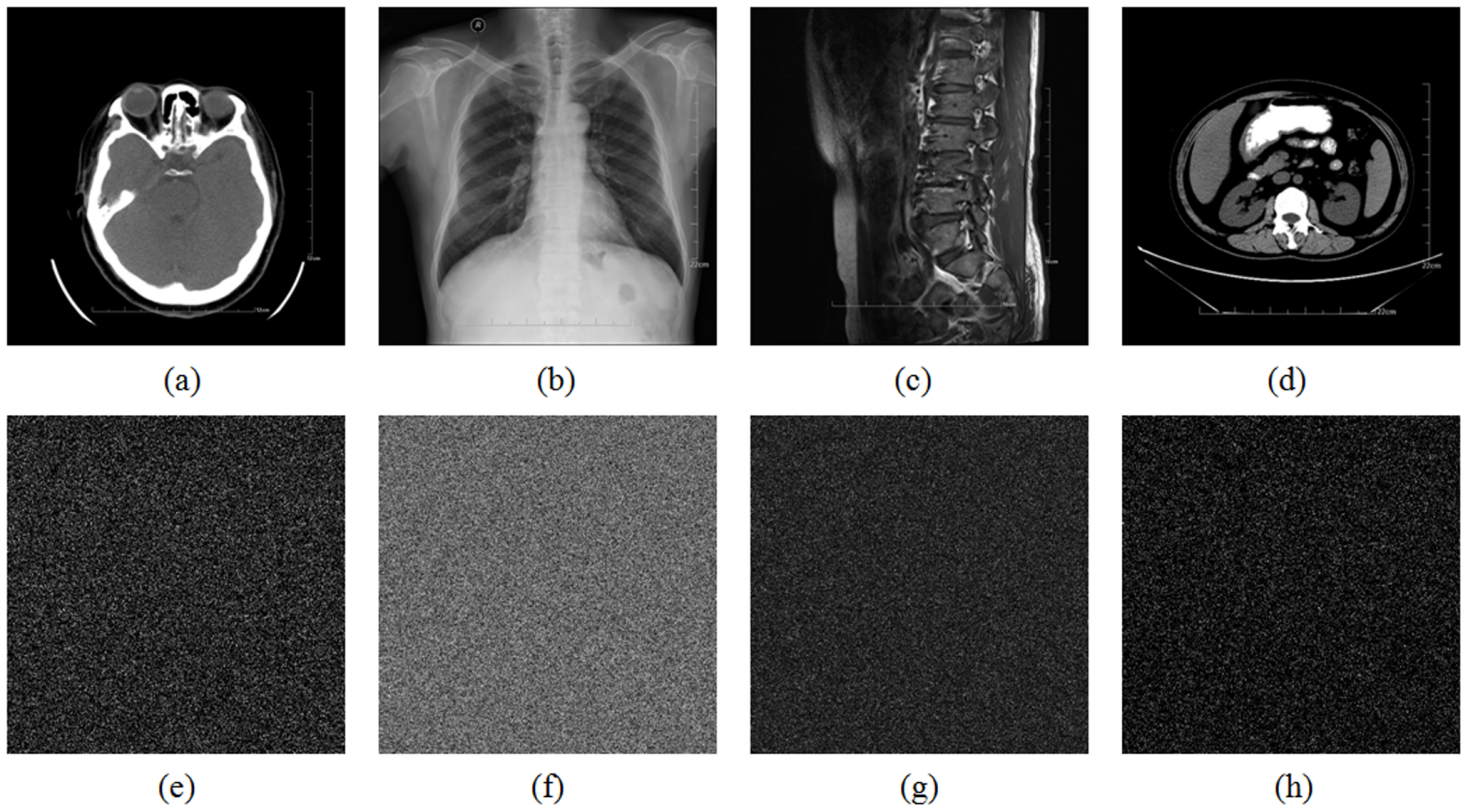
Test images and their corresponding shuffled images produced by the proposed method: (a) paranasal sinus CT. (b) chest X-ray. (c) waist MR. (d) abdomen CT. (e) – (h) are the shuffled images corresponding to (a) – (d), respectively.
